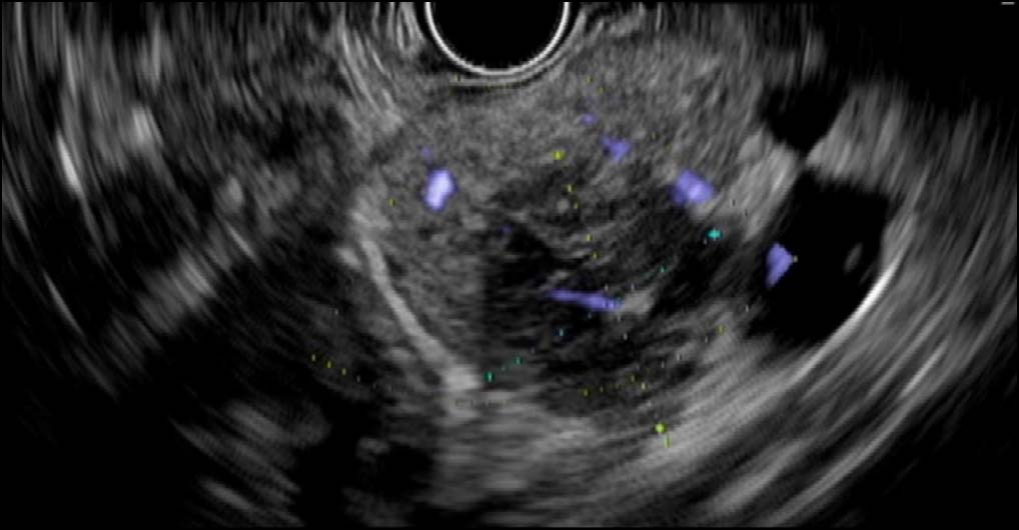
Endoscopic ultrasound demonstrating a 2.8 cm by 2.7 cm heterogeneous mass with irregular margins in the pancreatic body.
